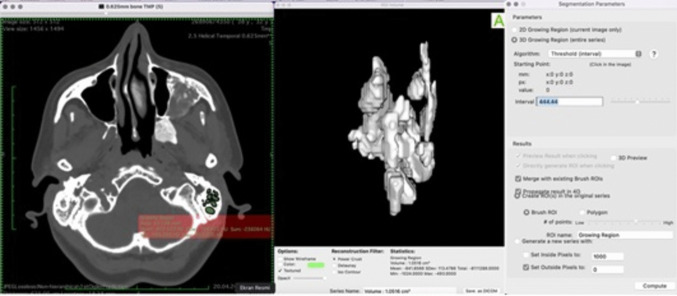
Procedure steps of measurement of mastoid pneumatization. Axial slice of the computed tomography scan of mastoid cells
